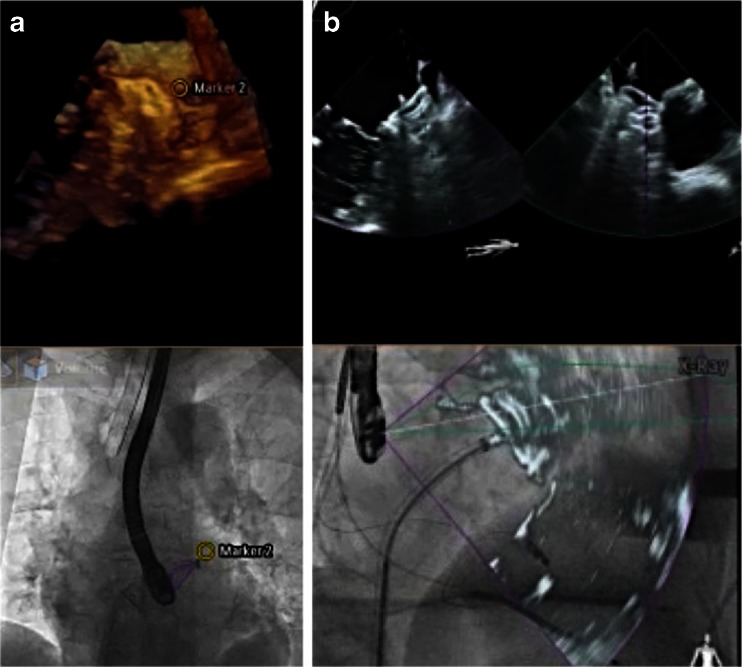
Fusion imaging during percutaneous left atrial appendage closure. a A marker in the echo image indicates the landing zone in the left atrial appendage, with the corresponding marker in the fluoroscopy image. b xPlane echocardiography (*top*) images demonstrate proper device placement in two planes, while simultaneous overlay imaging (*bottom*) ensures correct deployment and orientation of the (still attached) Amplatzer Cardiac Plug ® at the target zone
